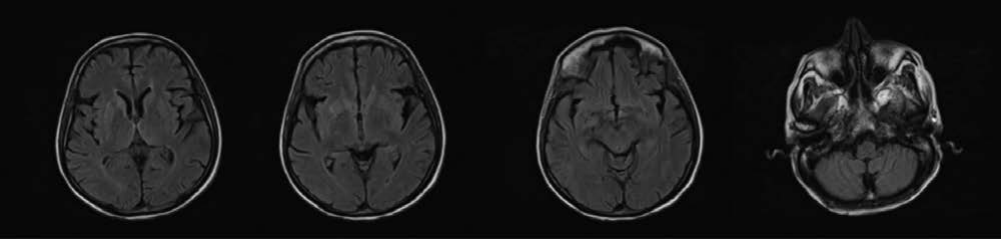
FLAIR MRI imaging shows that previous hyperintensities were almost completely resolved 1 month after thiamin replacement. FLAIR = fluid attenuated inversion recovery, MRI = magnetic resonance imaging.
